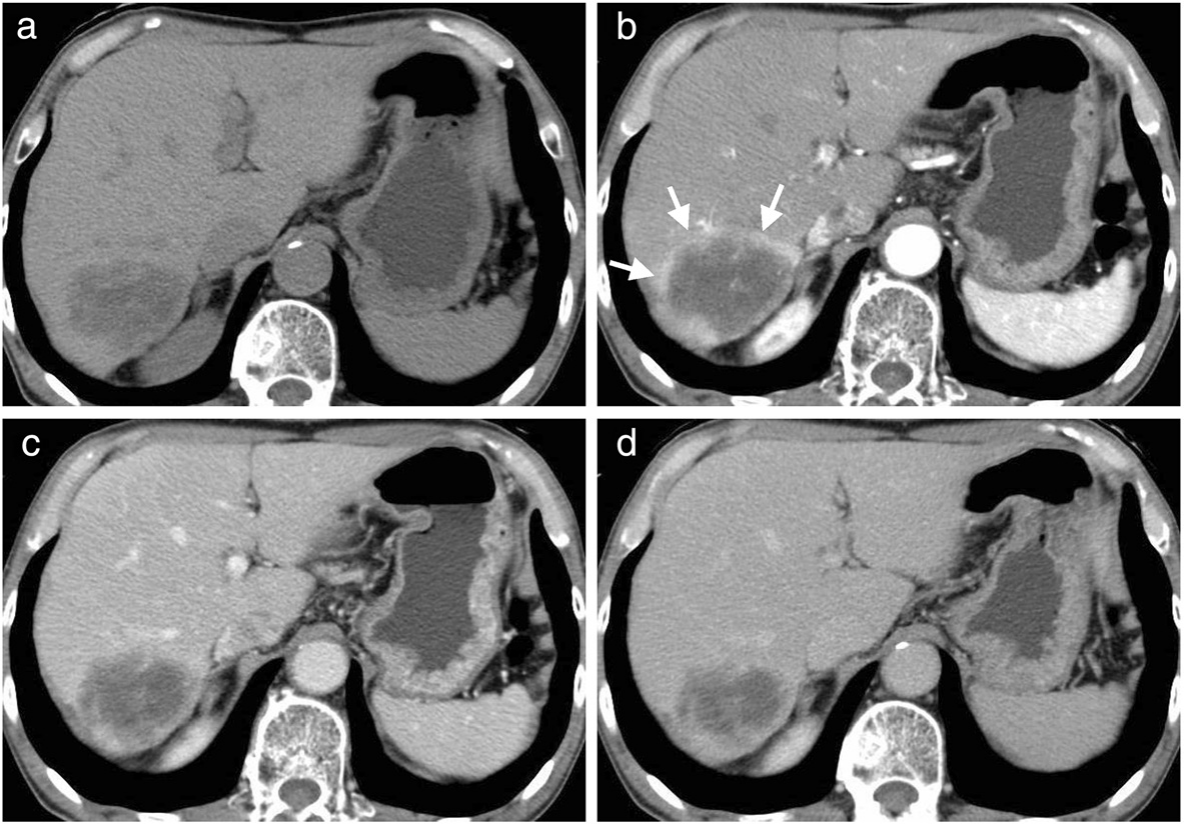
Abdominal computed tomography (CT). (a) plain, (b) arterial phase, (c) portal venous phase, and (d) delayed phase. Plain CT showed a low-density mass. Dynamic CT showed the ring enhancement in the peripheral part on the arterial phase (arrow).
